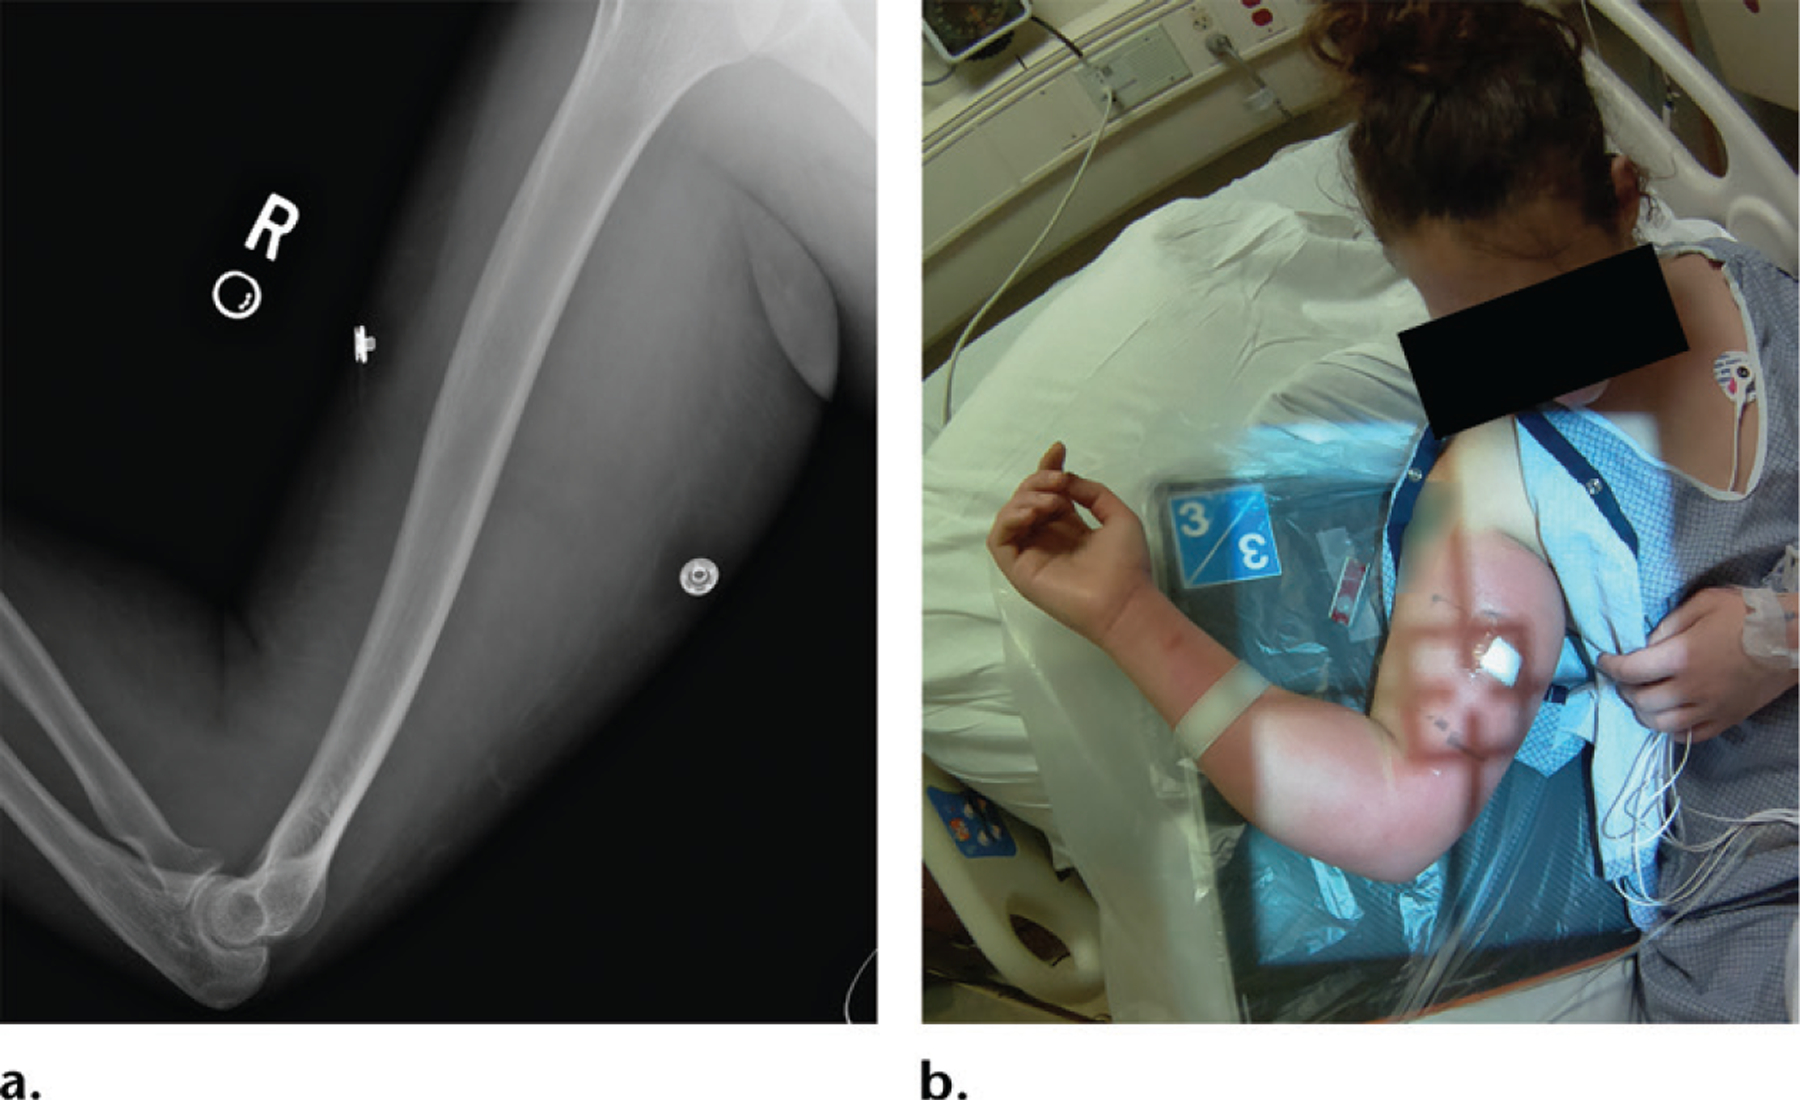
Radiograph (a) and patient photograph (b) show the right humerus in a woman with pain. Soft-tissue swelling was not noted at the initial evaluation of the radiograph but was noted on the final report after the radiologist integrated information obtained from the photograph. The soft-tissue swelling and erythema, findings consistent with cellulitis, were not noted in the provided clinical history. However, these findings are obvious on the photograph. This information could prompt the radiologist to evaluate for osteomyelitis rather than a fracture.
